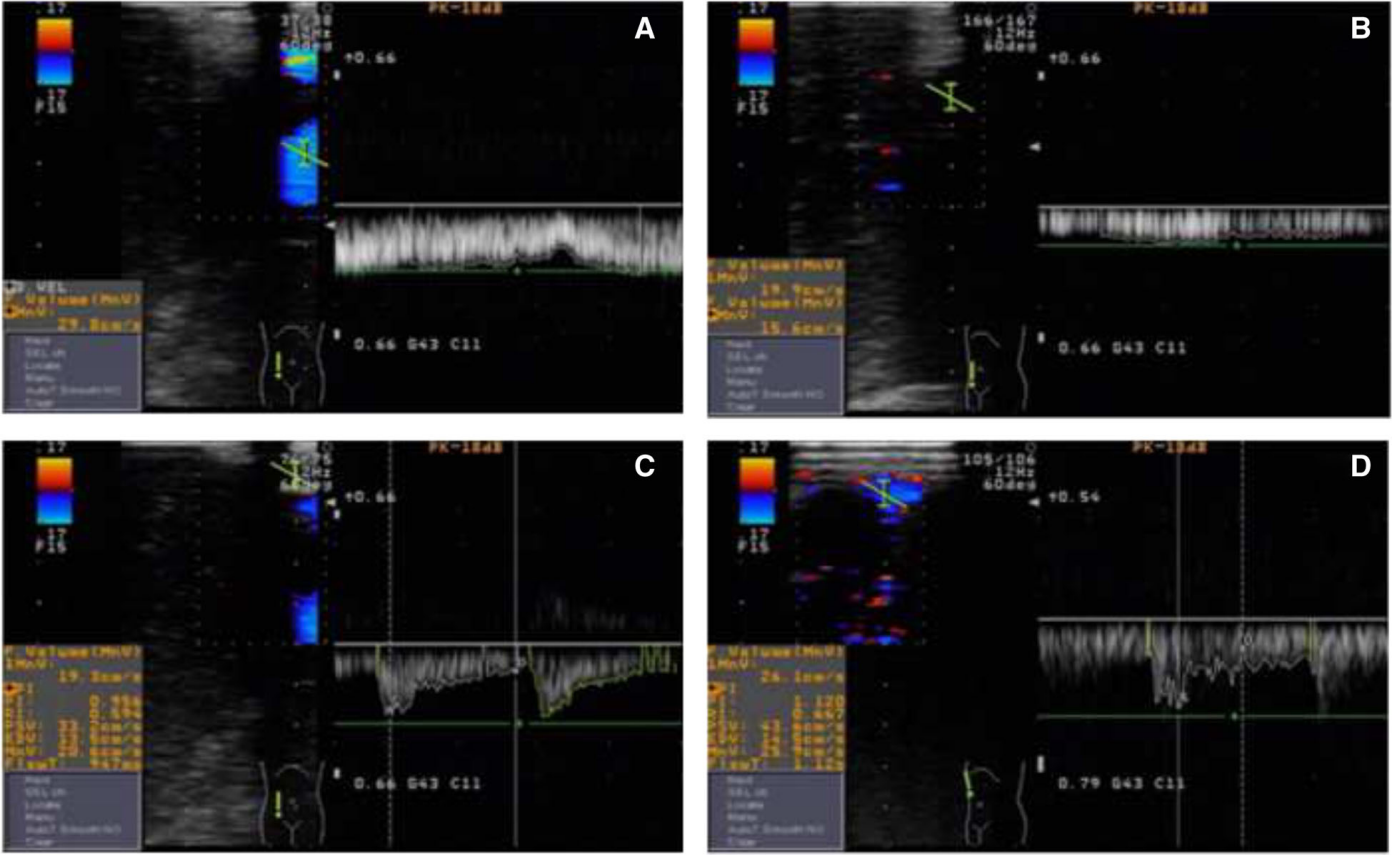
Morphologic changes in the hepatic blood flow induced by CO_2_-pneumoperitoneum on laparoscopic intraoperative ultrasonography. a Two-dimensional image of portal vein before insufflation of CO_2_. b Thirty min after insufflation of CO_2_ in the Trendelenburg position, the portal vein showed a marked decrease in the VD from 0.90 cm to 0.64 cm, as well as a marked decrease in MV from 29.50 cm/s to 14.60 cm/s. The calculated BFV decreased from 1126 ml/min to 281 ml/min. c Two-dimensional image of hepatic artery before insufflation of CO_2_. d Thirty min after insufflation of CO_2_ in the Trendelenburg position, the MV of hepatic artery was significantly increased from 19.2 cm/s to 26.1 cm/s with the VD less affected. The calculated BFV was similar (93 ml/min vs 96 ml/min) to that before insufflation of CO_2_. *VD* vessel diameter, *MV* mean flow velocity, *BFV* blood flow volume
